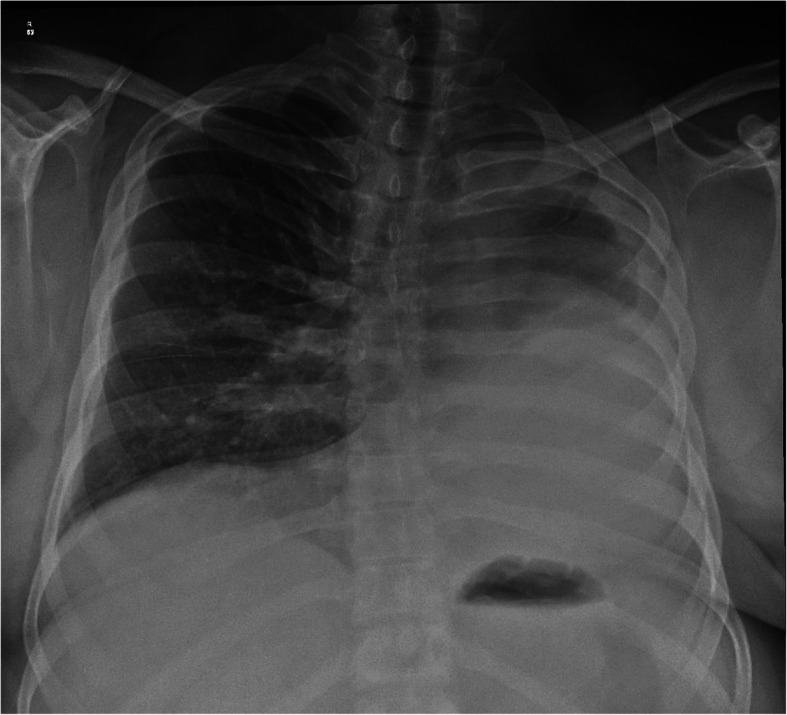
Chest radiograph in frontal projection showing homogenous opacity of left hemithorax with mediastinal shift and contralateral herniation of right lung into left upper hemithorax
